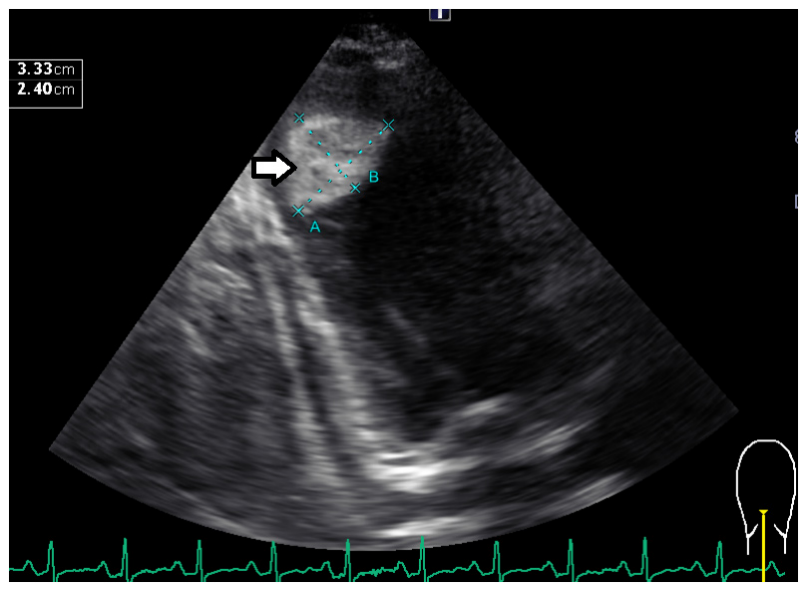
Echocardiogram (Apical 2 chamber view) showing dilated left ventricle with apical thrombus measurements.
